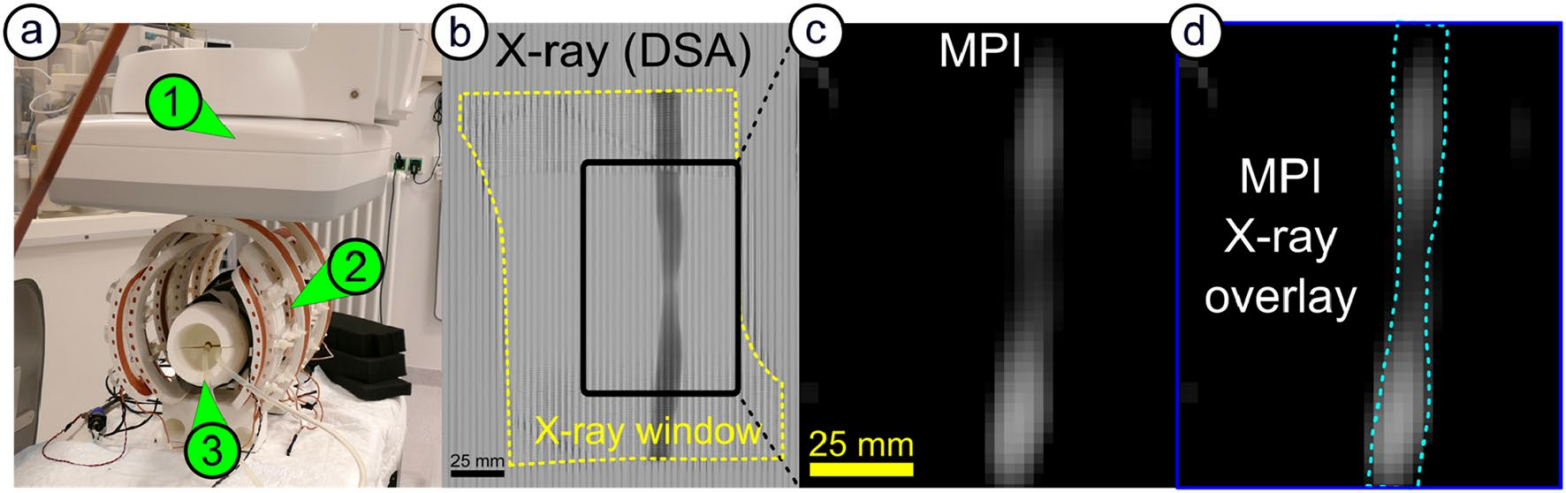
First simultaneous iMPI X-ray bolus tracking using a Perimag and iodinated contrast media mixture (ratio undiluted Perimag to iodined contrast agent of 1:1). (a) Image of the iMPI scanner (2) within the X-ray system (1). Simultaneous real-time visualization of the bolus through an artificial vascular stenosis in a human-size knee model (3): (b) X-ray and (c) iMPI. (d) shows an overlay of both modalities. For video see Supplementary file iMPImeetsXRay.mp4.
